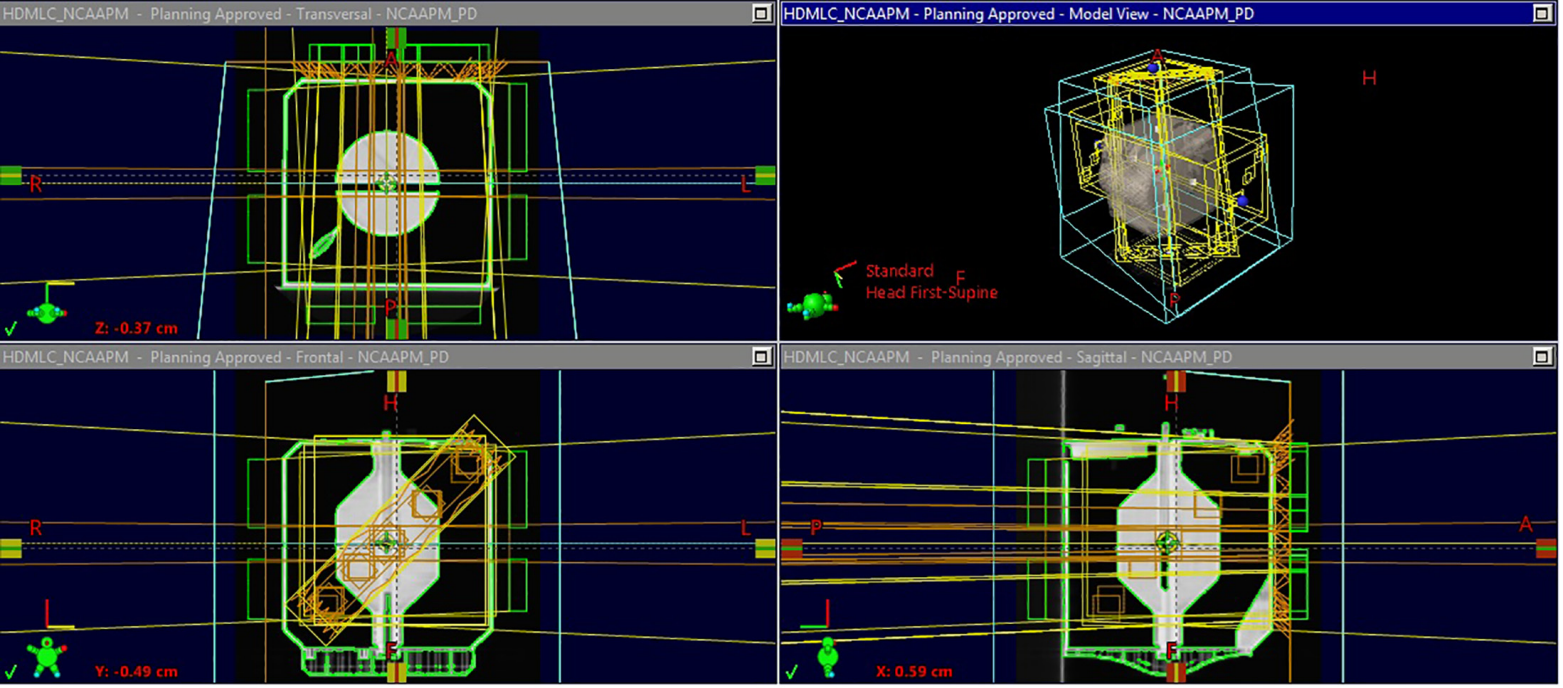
CT scan of OneIso and Off-axis Winston-Lutz beam geometry.
